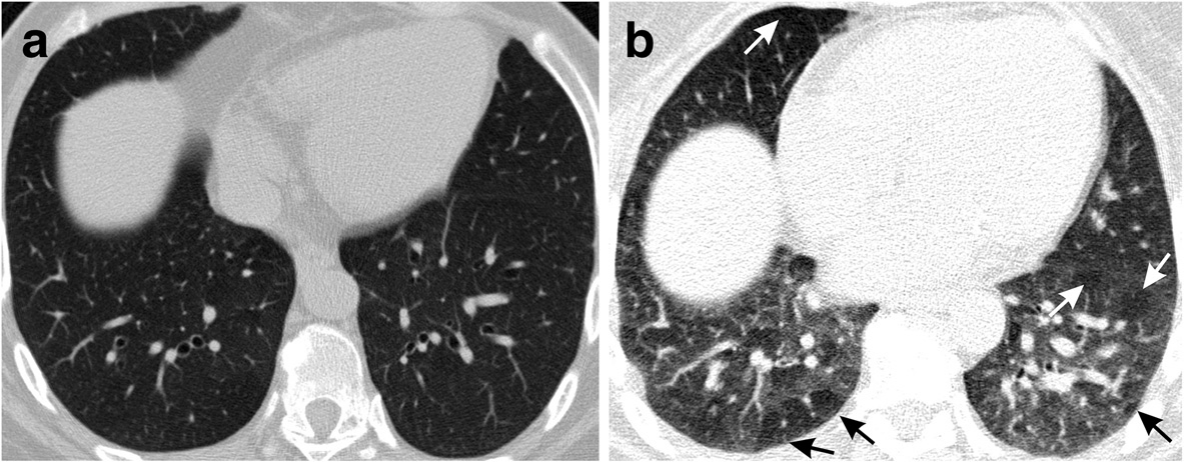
Air trapping in a 57-year-old man with acromegaly who had a RV value of 173% of predicted and a Raw value of 3.47 cm H_2_O/L/s. (a) Inspiratory, transverse, thin-section CT scan obtained through the lower lung zone shows no abnormal findings. (b) Expiratory, transverse, thin-section CT scan obtained through the lower lung zone reveals diffuse air trapping (arrows).
